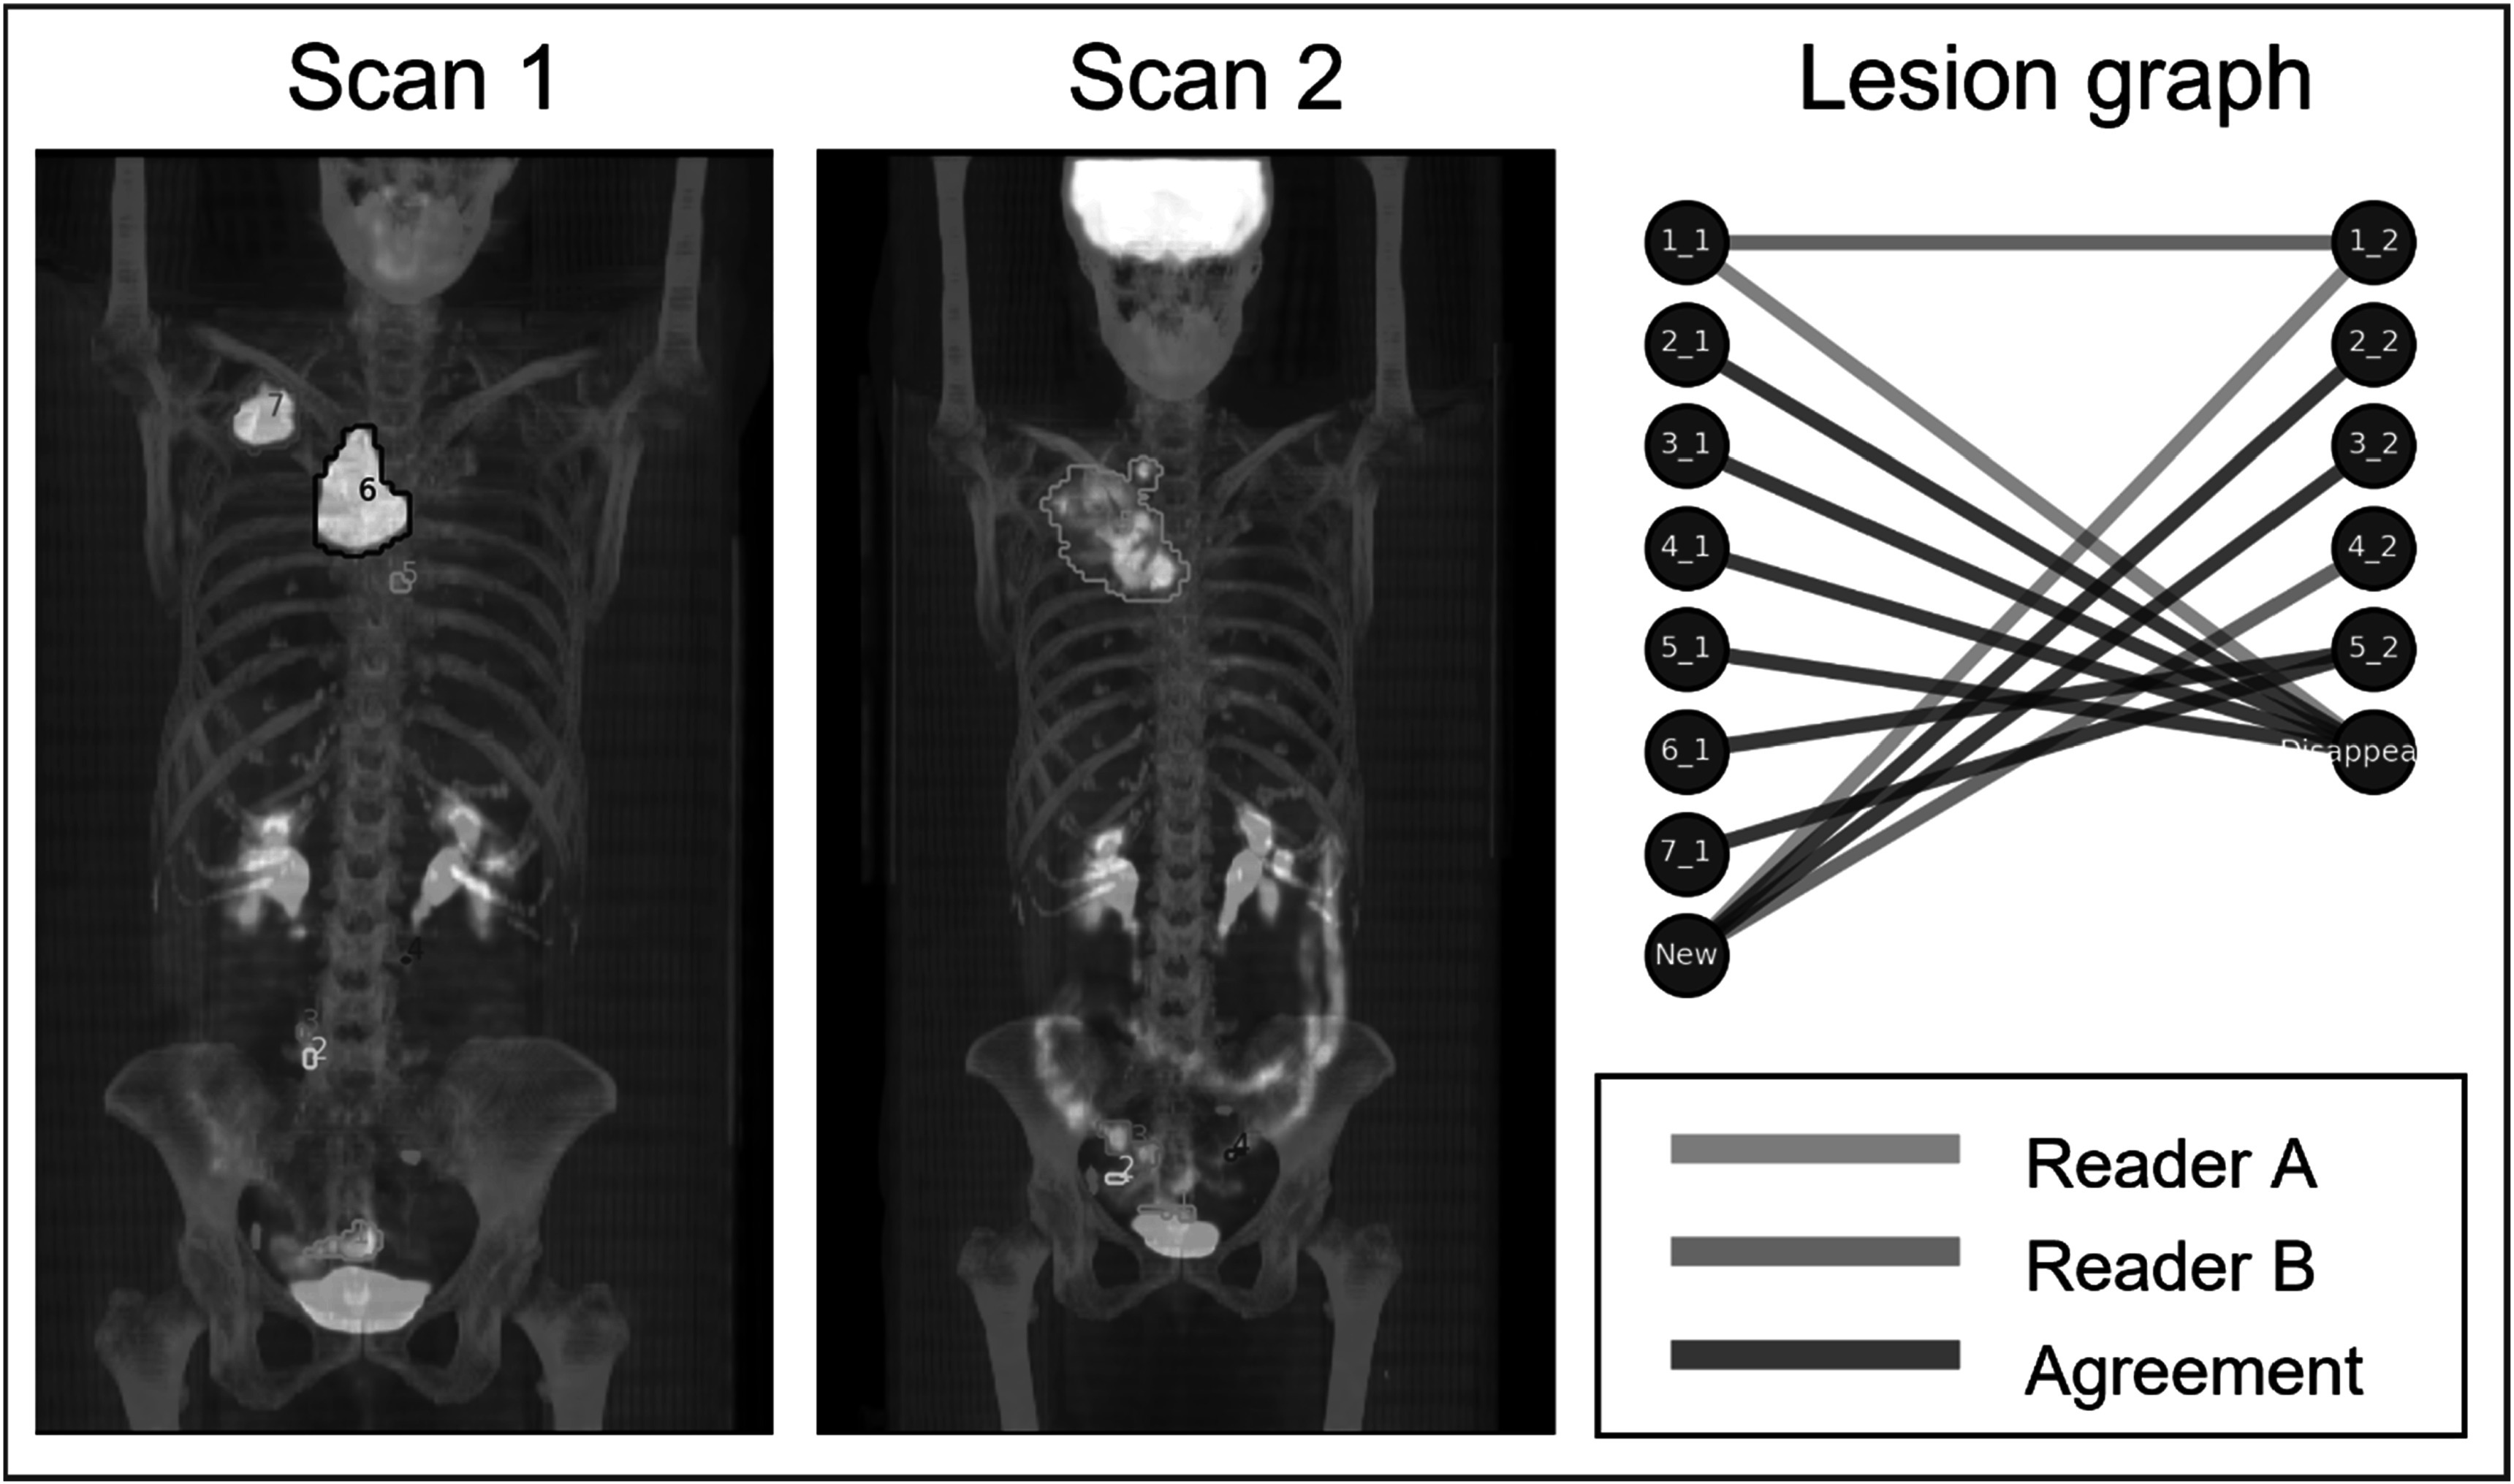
Lesion matching in a female subject with stage III NSCLC imaged with ^18^F-FDG PET/CT before (Scan 1) and after (Scan 2) platinum-based chemotherapy. PET/CT were acquired 179 d apart. The lesion located in the rectum (orange contour, label 1) is matched differently between readers. Reader A determines that the lesion disappears after scan 1 and a new lesion in a similar area appears on scan 2. Reader B determines that these two lesions are homologous and should be matched between scans.
